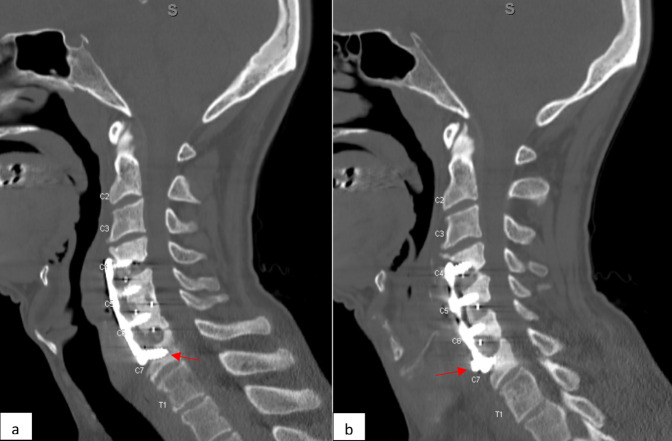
CT of the cervical spine 7 years after ACDF demonstrating (A) nonunion of C4-5 and C6-7 with a lucency around the left C7 screw and (B) 3-mm pullout of right C7 screw. ACDF = anterior cervical diskectomy and fusion
